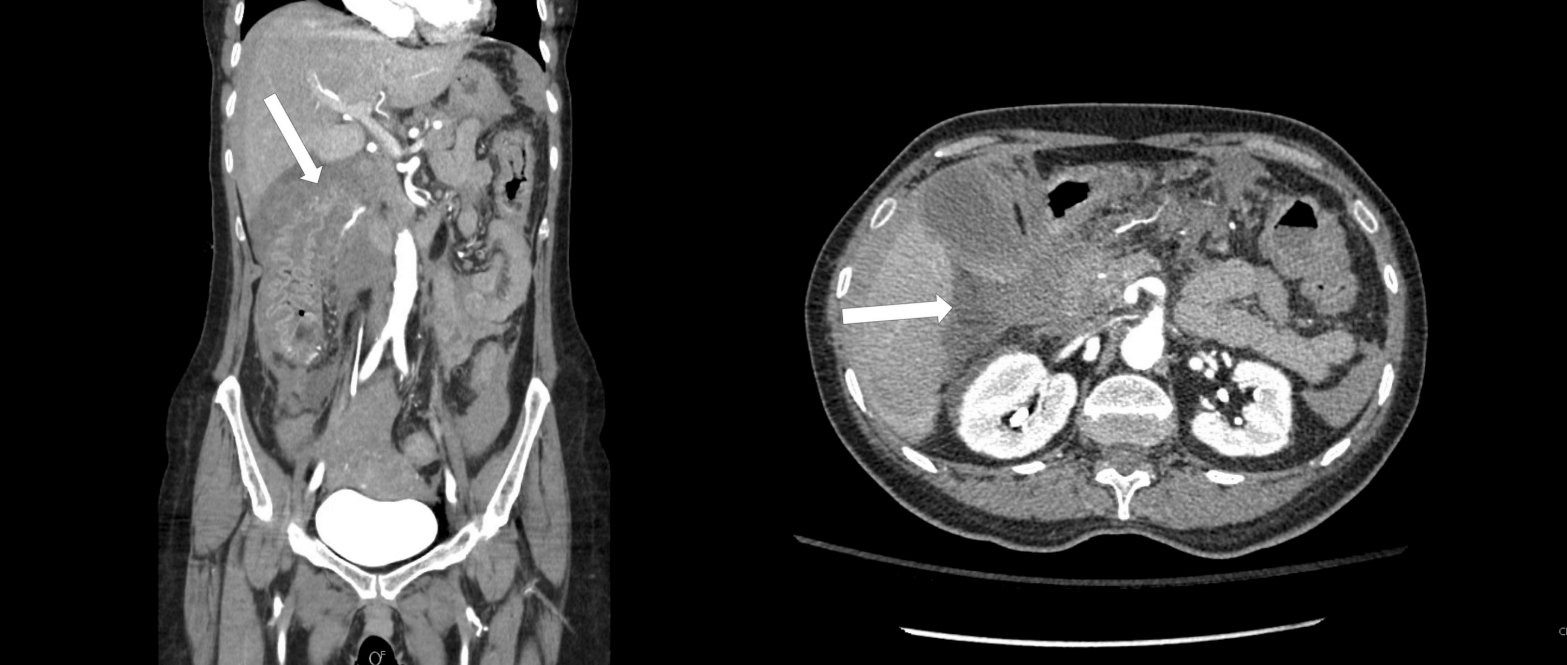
Axial CT angiography of the abdomen and pelvis demonstrating a pseudoaneurysm with active contrast extravasation from a mesenteric branch of the superior mesenteric artery (arrow).
